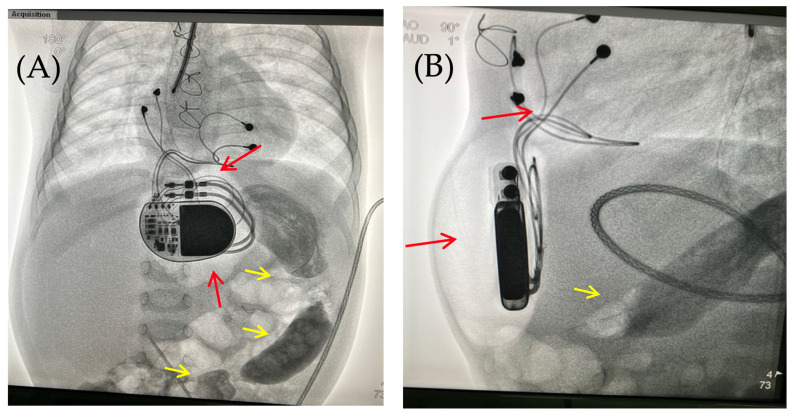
Chest X-ray examination—frontal (A) and lateral (B) view. Chest X-ray shows no pneumothorax, but pneumopericardium, pneumomediastinum with air are present at the pacemaker pocket site (red arrow). Radiocontrast agent (yellow arrow) at the gastrointestinal tract.
